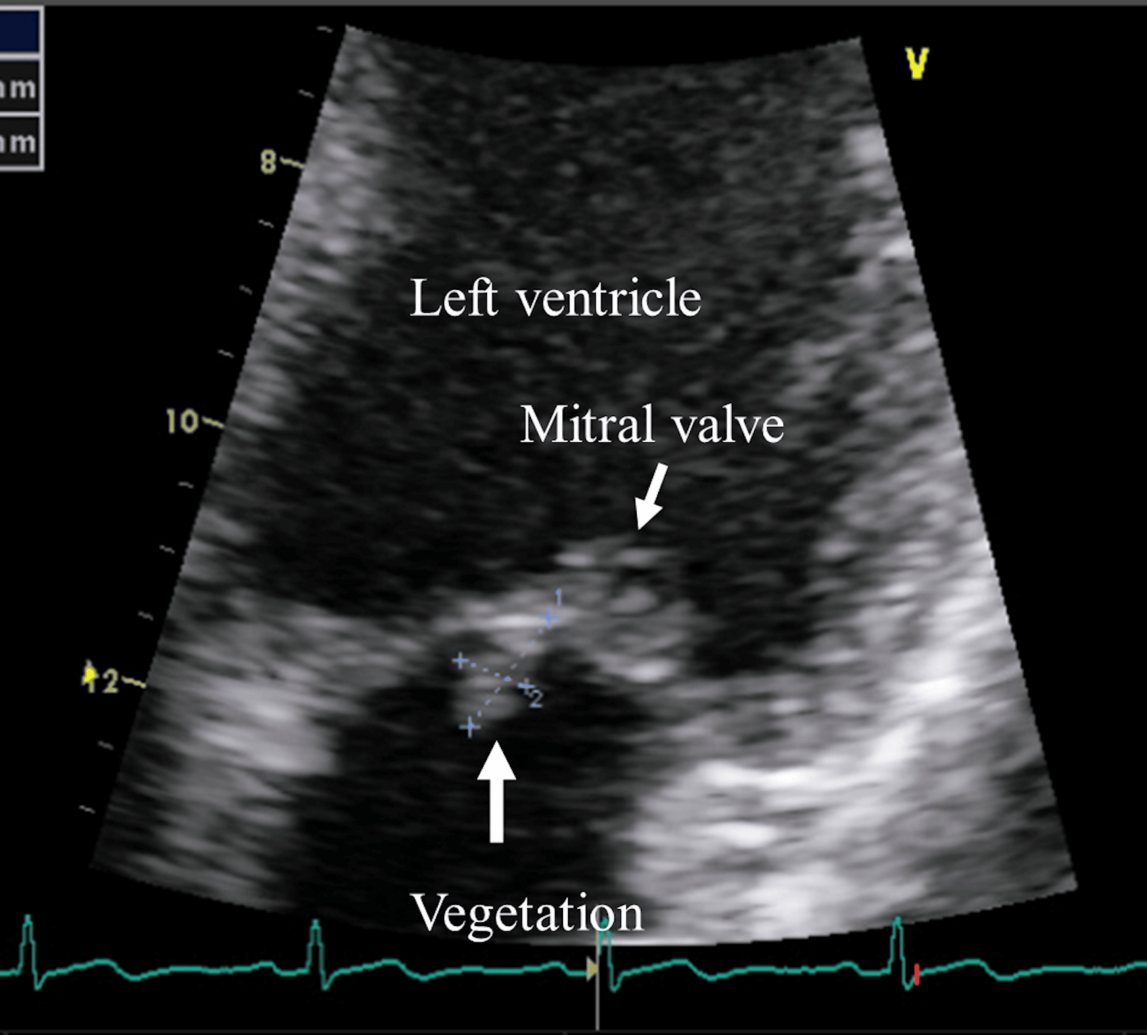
Mitral valve vegetation identified by transthoracic echocardiography A 9.9/5.2 mm nodular, hyperechogenic vegetation attached to the anterior mitral leaflet was revealed.
